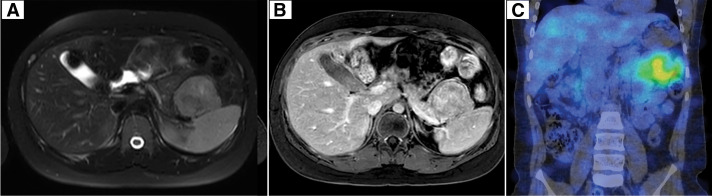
Abdominal MRI and somatostatin receptor scintigraphy. (A) Abdominal MRI revealed a T2-hyperintense 6-cm solid lesion in the anterior of the pancreatic tail. (B) Contrast-enhanced MRI revealed the T1-hypointense tumor with a rim of contrast enhancement. (C) Somatostatin receptor scintigraphy with ^111^In-pentetreotide (OctreoScan) showed homogeneous radiotracer uptake in the tumor.
